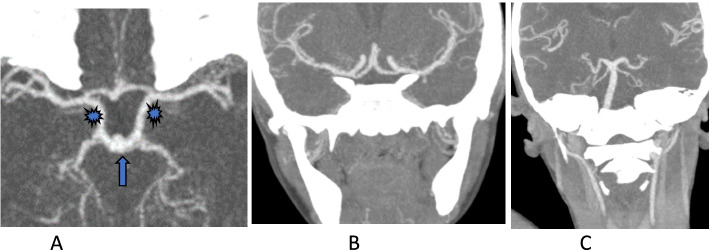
Brain arteries with corresponding almost equal segments look like “normal” circle of Willis; Anterior circulation is supplied through the prominent posterior circulation including dominant basilar (arrows) and posterior communicating arteries (asterisks) presenting on axial MIP CTA (A) and also on reformatting coronal MIP CTA images (B, C,). Abbreviations: CTA- Computed Tomography Angiography; MIP- maximum intensity projection
